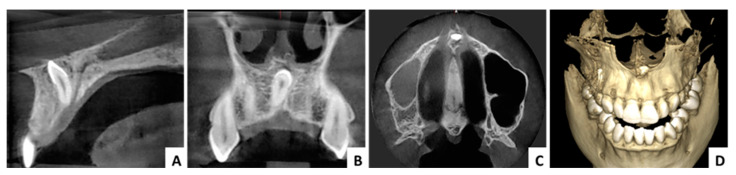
Case 1—radiological aspects: (A) CBCT cross-sectional view, (B) CBCT panorama view, (C) CBCT axial view, (D) CBCT 3D reconstruction.
